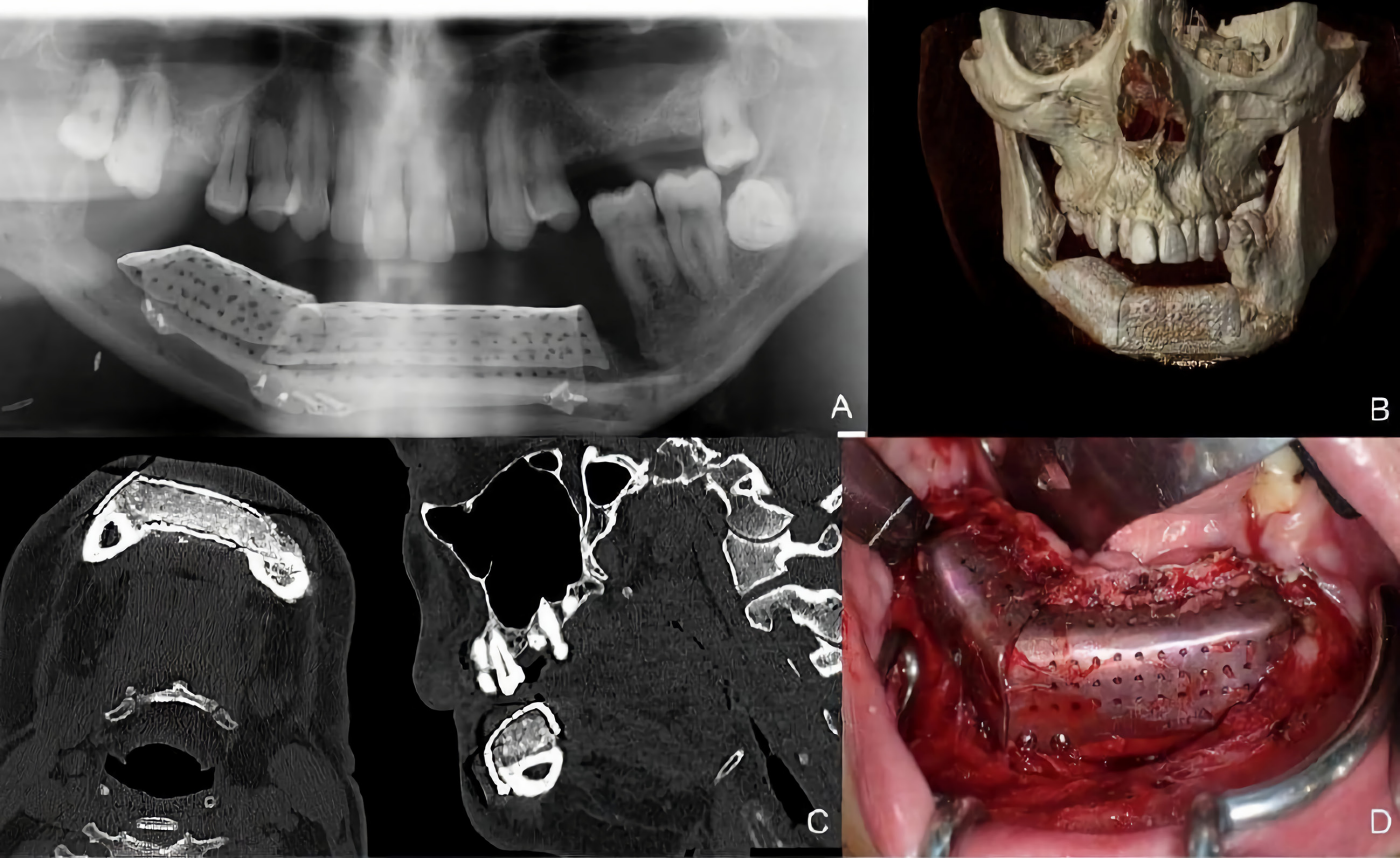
(A) Panoramic radiograph showing a bone gap between the iliac crest graft and the remnant mandible mesially to the lower molar due to the angular shape of the titanium mesh at this level. CT Scan (B) with three-dimensional reconstruction of the mandible. CT Scan demonstrating the stability of the transverse dimension of the fibula with respect to the remnant mandible. (C) The three-dimensional preservation of the iliac crest graft with CAD/CAM mesh makes it possible to double the height of the fibula. (D) Intraoral approach showing the three-dimensional preservation of the customized mesh.
